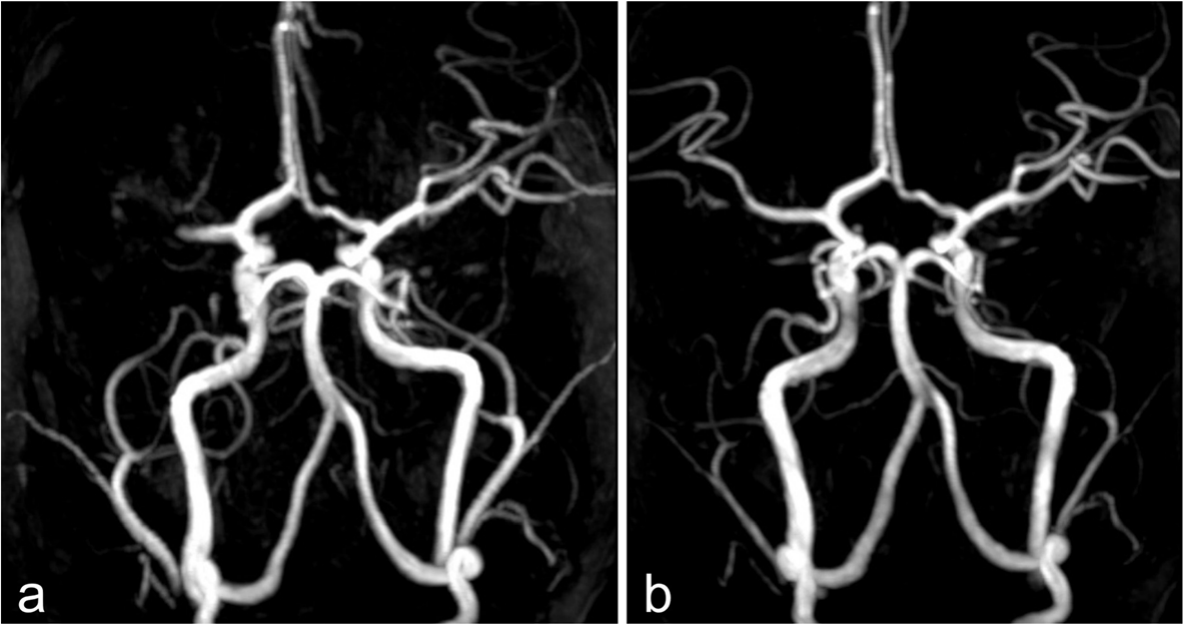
Cerebral arteriogram showing a right-sided middle cerebral artery infarction (a). Following thrombolytic therapy with enoxaparin, the MRI revealed normal perfusion of the cerebral arteries (b).
